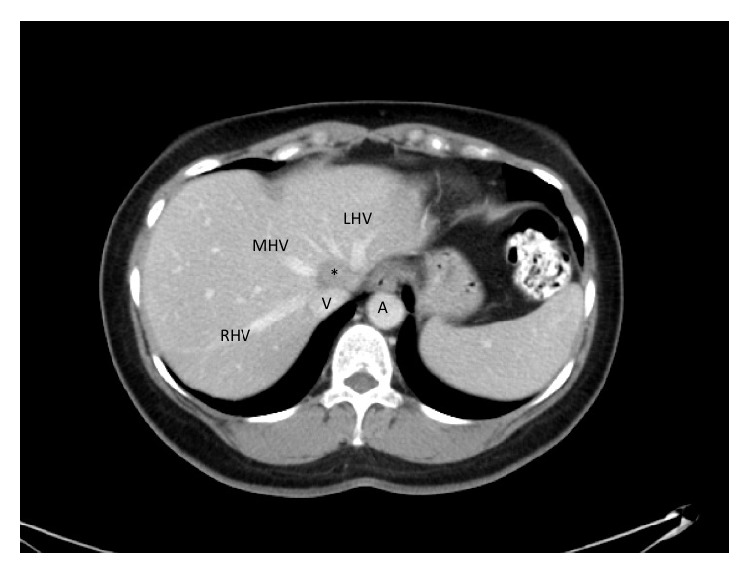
Axial CT images showing the IVC (V) receiving the LHV, MHV, and RHV. The solitary metastatic deposit (∗) is seen wedged between MHV and LHV and encroaches on the IVC. On axial cuts, the metastatic deposit is juxtaposed to, but does not appear to, involve the RHV.
